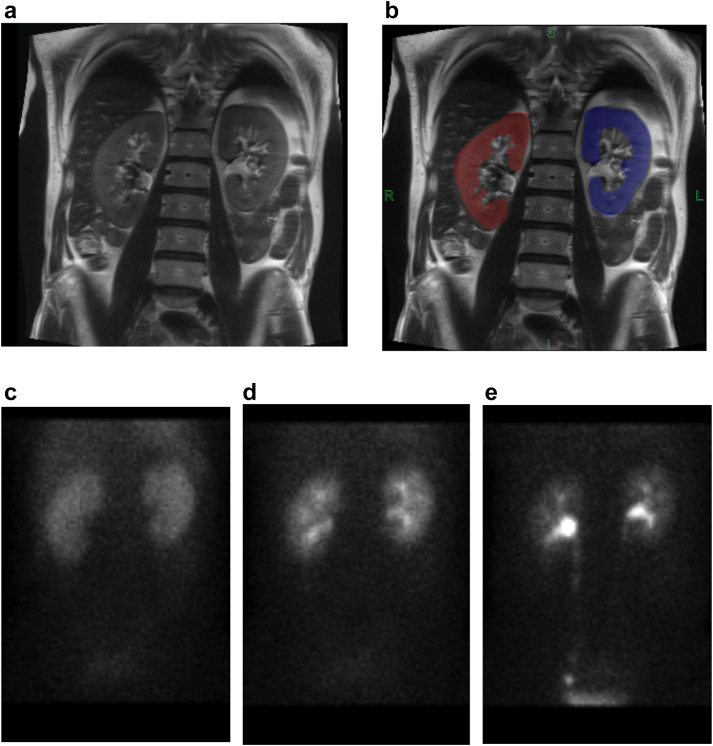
(a and b) Volume measurement from HASTE magnetic resonance imaging and (c) ^99m^Tc-DTPA dynamic planar scintigraphy (gamma camera) images for a renal mass patient in cortical, (d) medullary, and (e) urinary phases of clearance.
